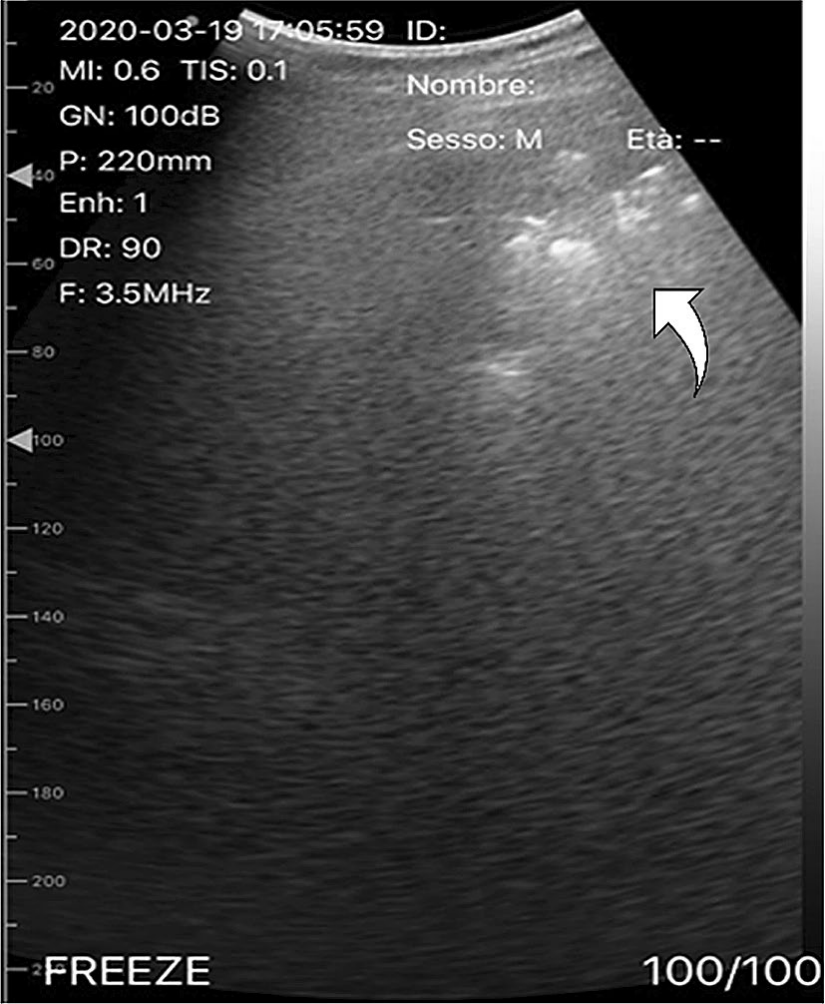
Pulmonary ultrasound pattern “wet” (the arrow indicates a consolidation with subpleural consolidations)
